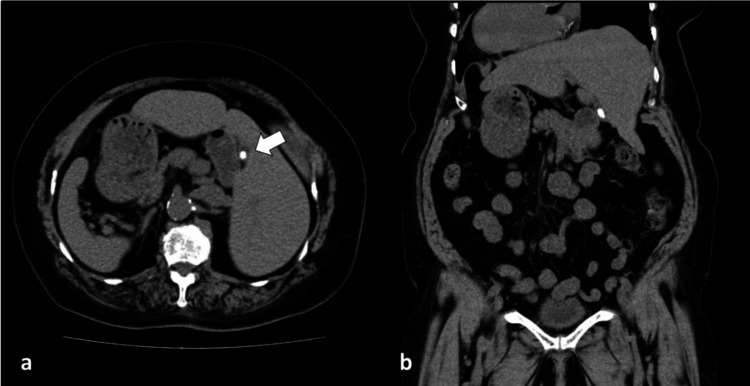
(a) Axial CT images revealing a 15-mm gallstone within the gallbladder (arrow), along with increased adipose tissue consistent with obesity. (b) Coronal CT images showing that all organs are arranged in a mirror-image configuration compared with the normal anatomy, consistent with situs inversus totalis CT: computed tomography
